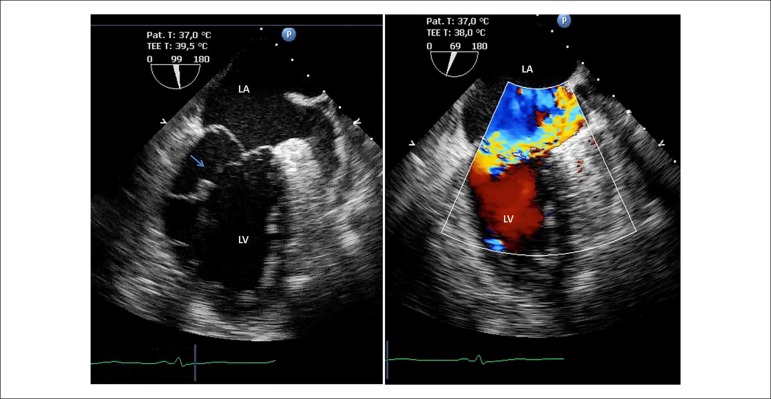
Transoesophageal echocardiogram two-chamber view showing a 9 mm
